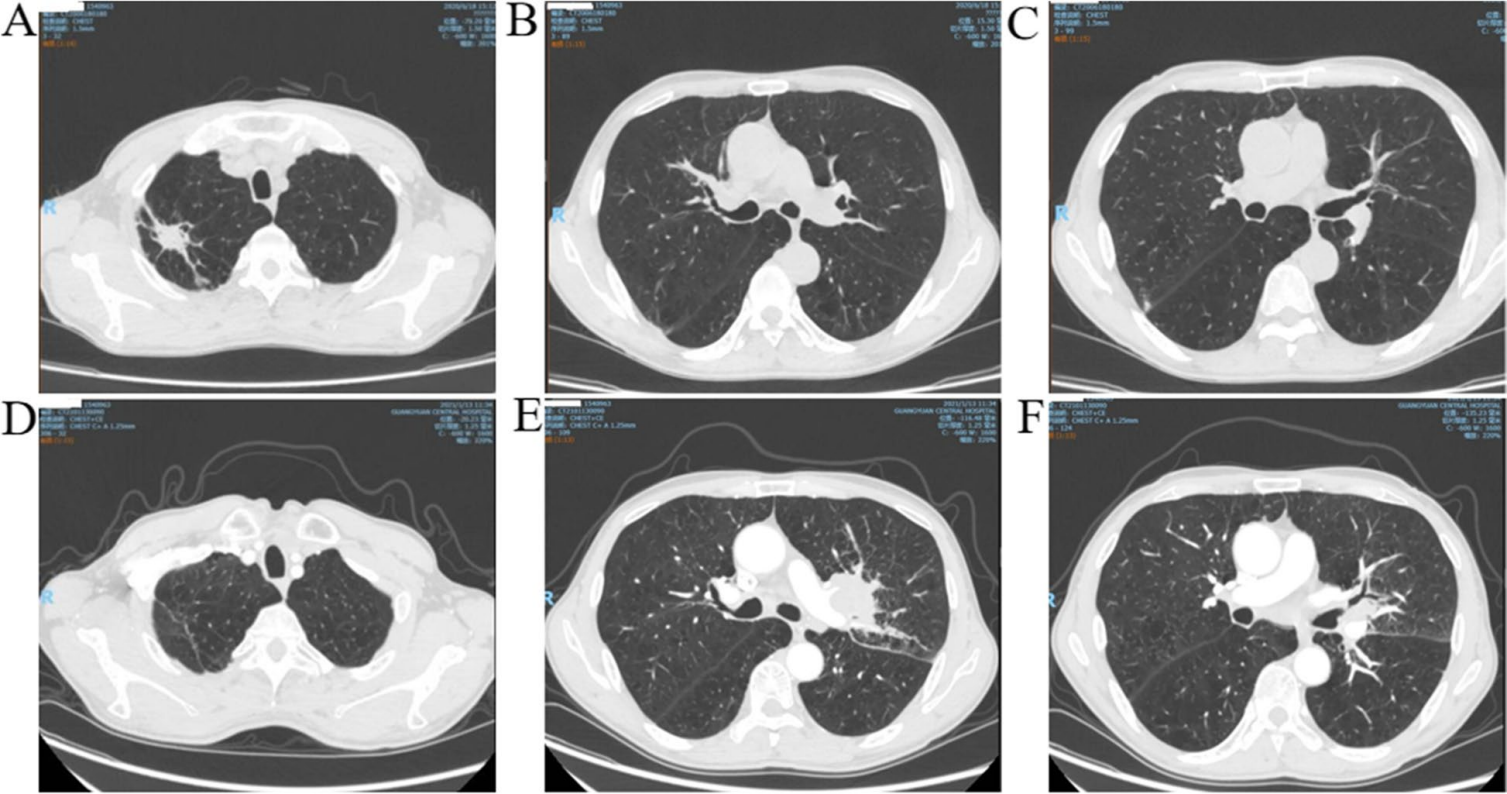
A, B, and C are the chest CT scan results from the first admission. D, E, and F show the results of chest CT scan at the same layer from the second hospitalization
